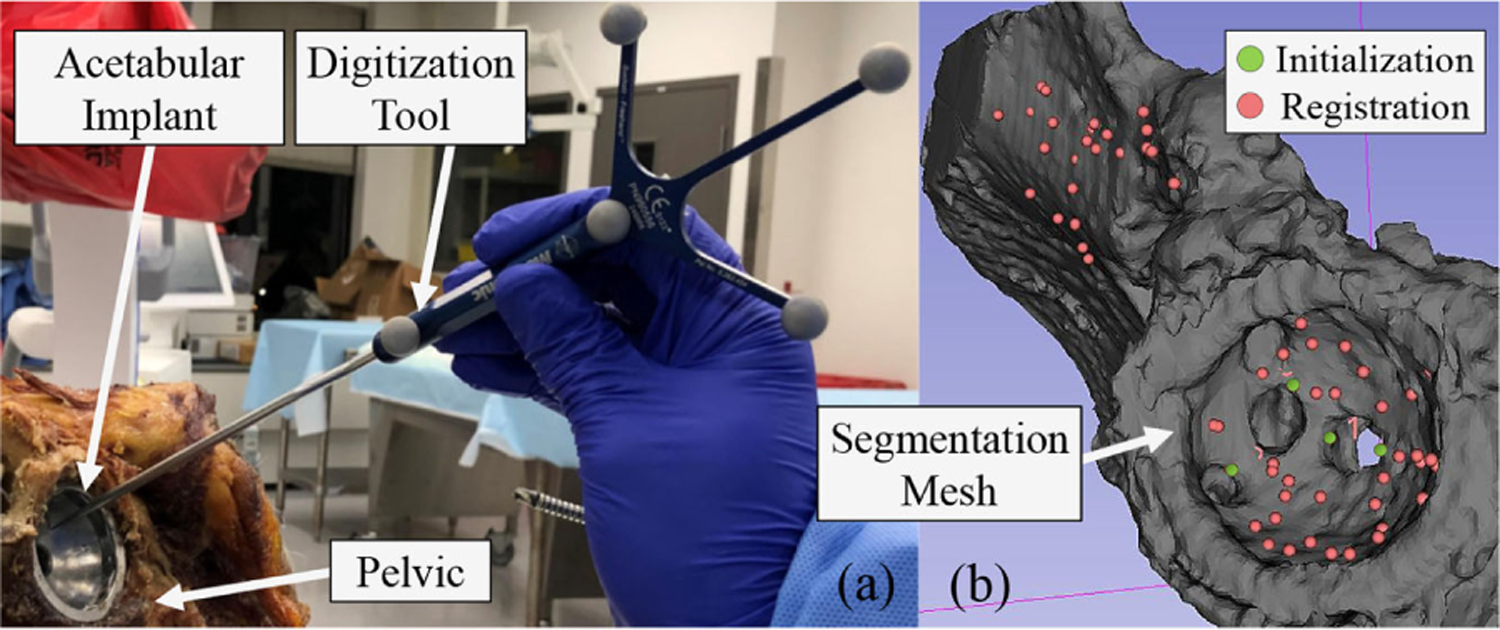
Registration procedure. (a) Digitization of the acetabular cup implant. (b) Overlayed digitized point cloud on the preoperative CT after registration.
